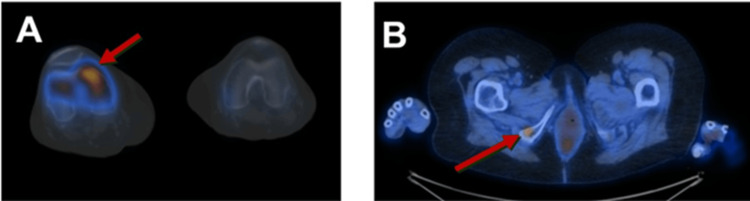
PET/CT scan showing hypermetabolic activity in the right knee (A) as well as a 7 mm hypermetabolic lesion in the right ischial tuberosity (B).
